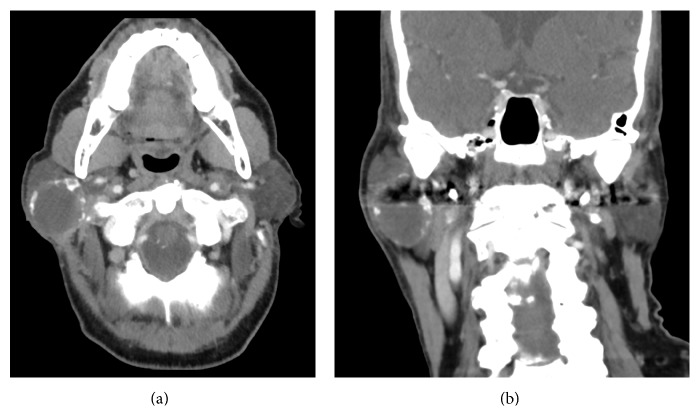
Computed tomography scan. (a) Axial and (b) coronal sections demonstrating a well-circumscribed, solid, isodense 4 × 3.5 cm mass with rim of peripheral calcification.
